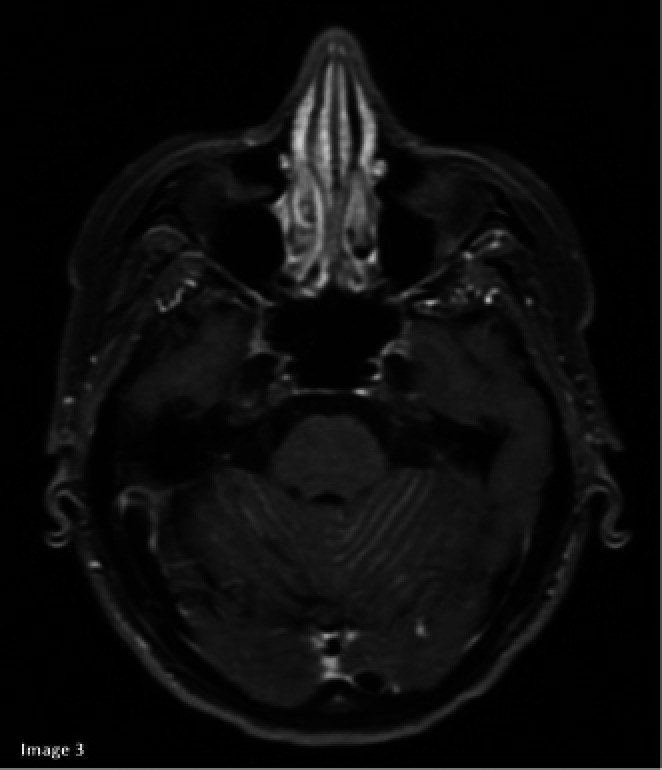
IMAGE 3 Axial T1‐weighted MRI showing showing leptomeningeal contrast enhancement of the cerebellar folia.
